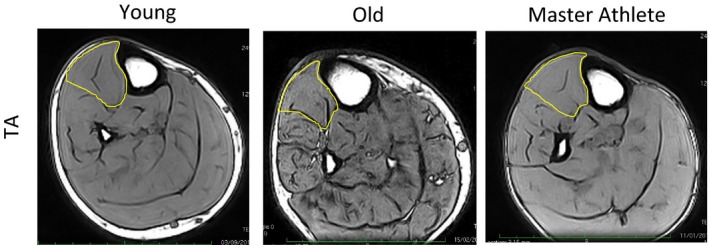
MRI images of the lower leg of Young, Old, and Master Athlete participants. The tibialis anterior (TA) muscles are outlined in yellow. These images were taken from the mid‐belly motor point of the TA.
